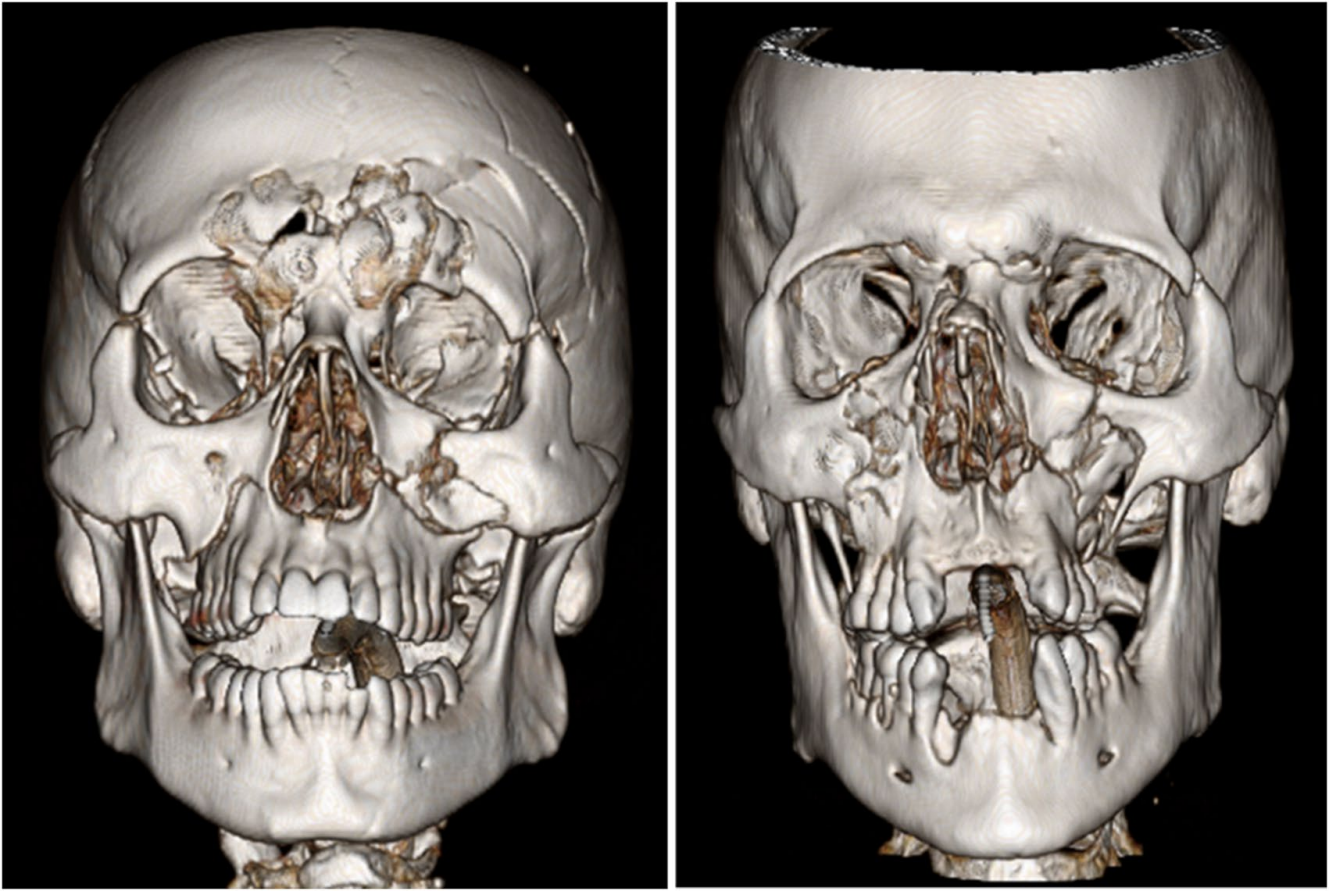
3D rendered CT images from the second pair of assessment cases. Both cases demonstrate LeFort, NOE, and frontal sinus fractures with different degrees of comminution and levels of involvement. These cases were randomized to pre- and post-lecture assessments to evaluate for improved reporting of these subunits
